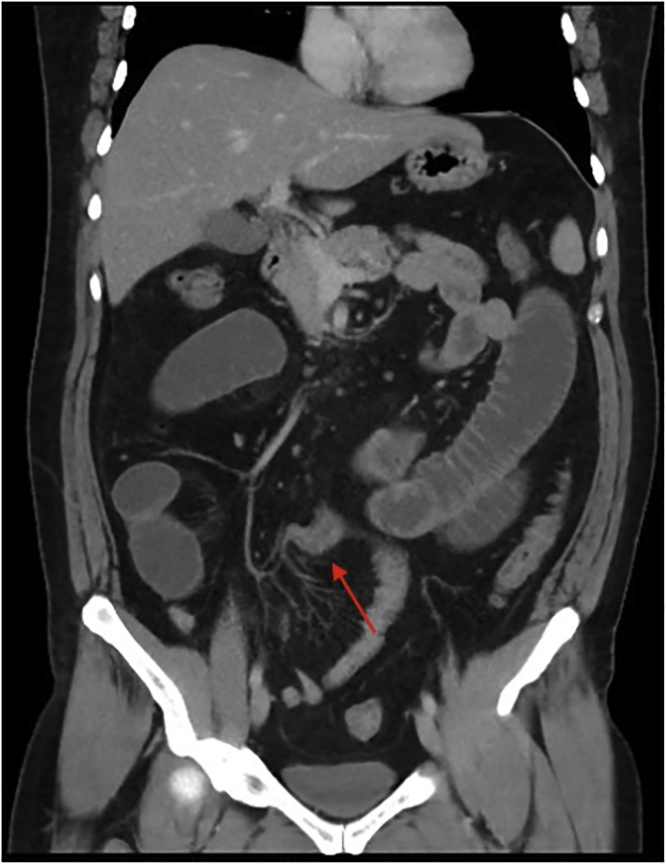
Coronal view of the CT abdomen and pelvis showing transition point of the SBO with the angulation of the small bowel suggesting a kink.
